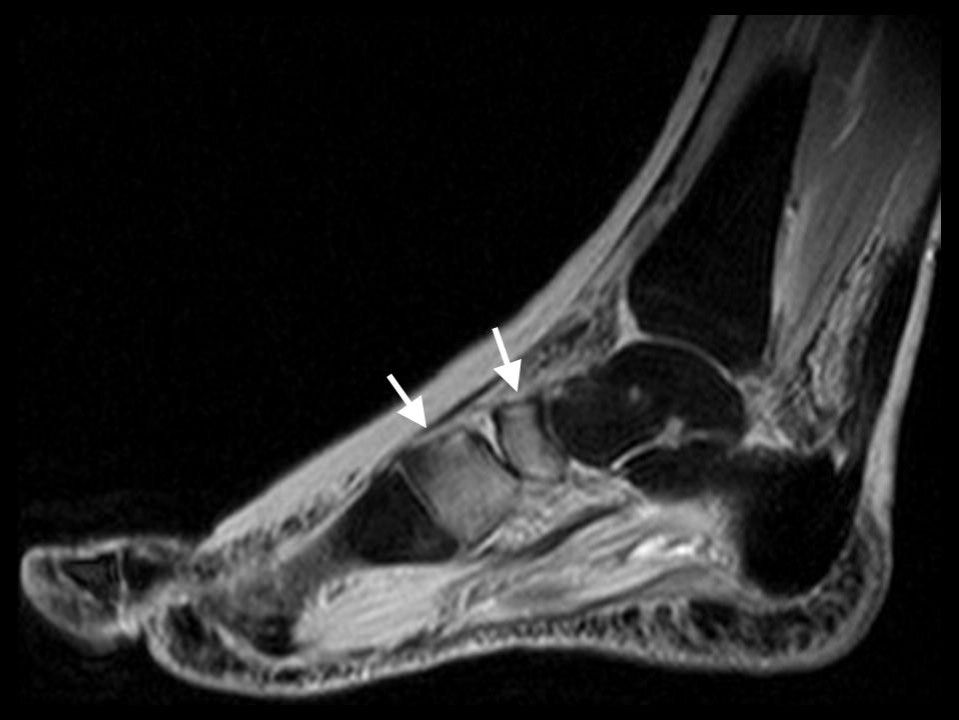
Same foot as in Figure 5. MRI sagittal STIR-sequence shows a circumscript edema with skin thickening at the midfoot and the anterior tarsus, with bone marrow edema in the navicular and medial cuneiform bones (white arrows), and edema of adjacent soft tissues (stress injury grade III).
